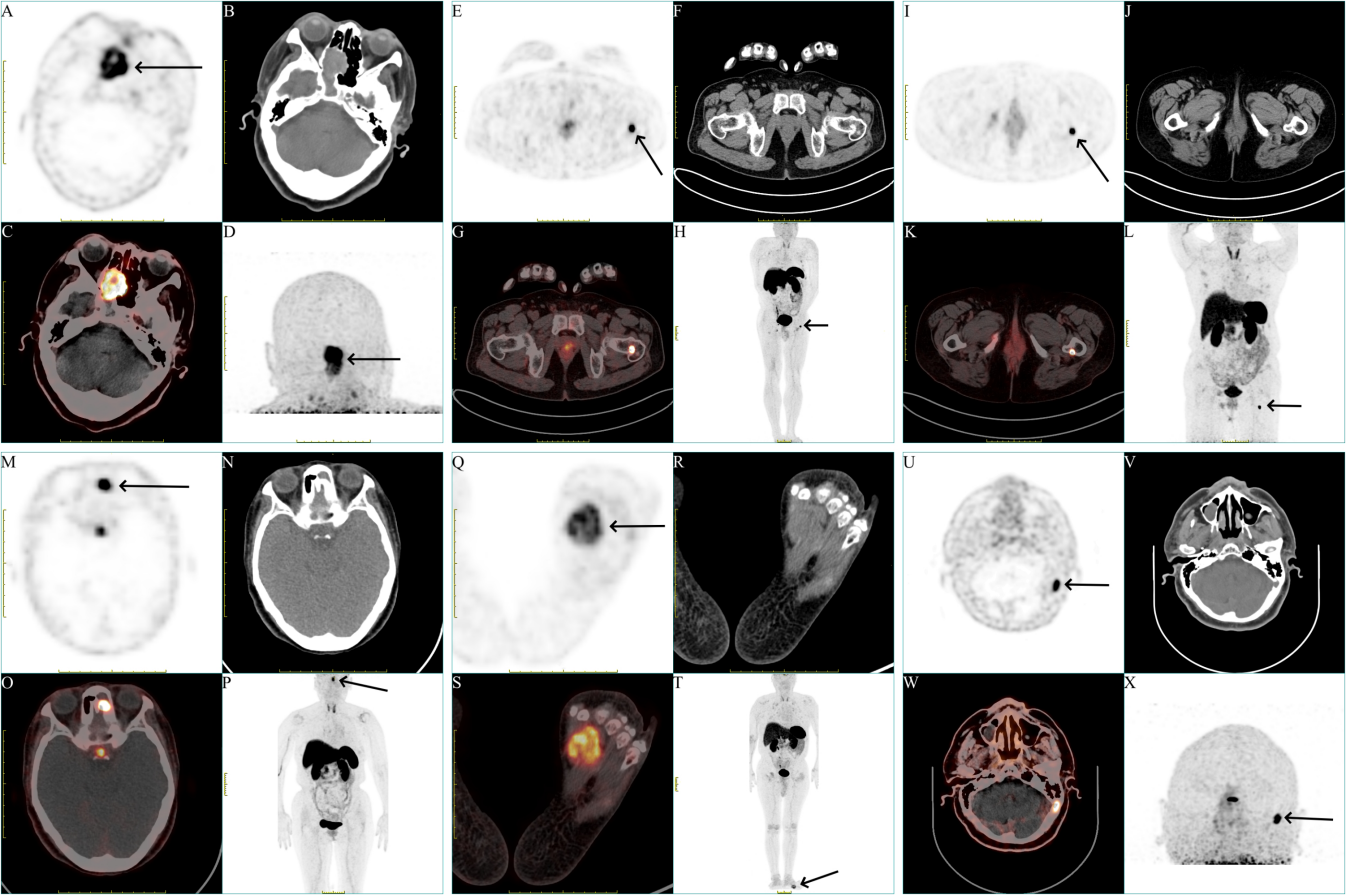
Representation of different locations of the tumors of all patients. (A, E, I, M, Q, U) Axial view of the tumors in ^18^F-OC PET (black arrow). (B, F, J, N, R, V) Axial view of the tumors in CT. (C, G, K, O, S, W) Fusion image. (D, H, L, P, T, X) Maximum-intensity projection (MIP) image of the patients (black arrow).
